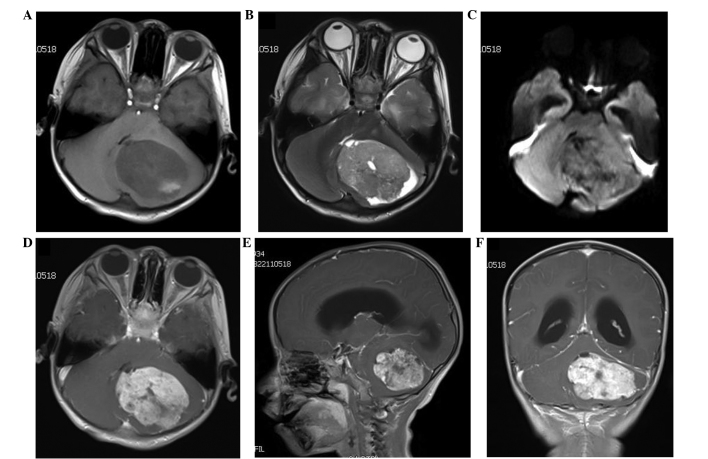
(A-D) Transaxial, (E) sagittal and (F) coronal magnetic resonance imaging prior to surgery, showing a large mass (40-mm maximum diameter). (A) On T1WI, the mass showed a relatively homogeneous uniform signal intensity, with patchy areas of high T1 signal and slight edema around the lesion. (B and C) The fourth ventricle was pushed to the right side and had become narrowed. The tumor exhibited a slightly increased signal intensity on T2- and diffusion-WI. (D–F) T1WI with gadolinium showed a well-demarcated, enhanced mass. WI, weighted imaging.
